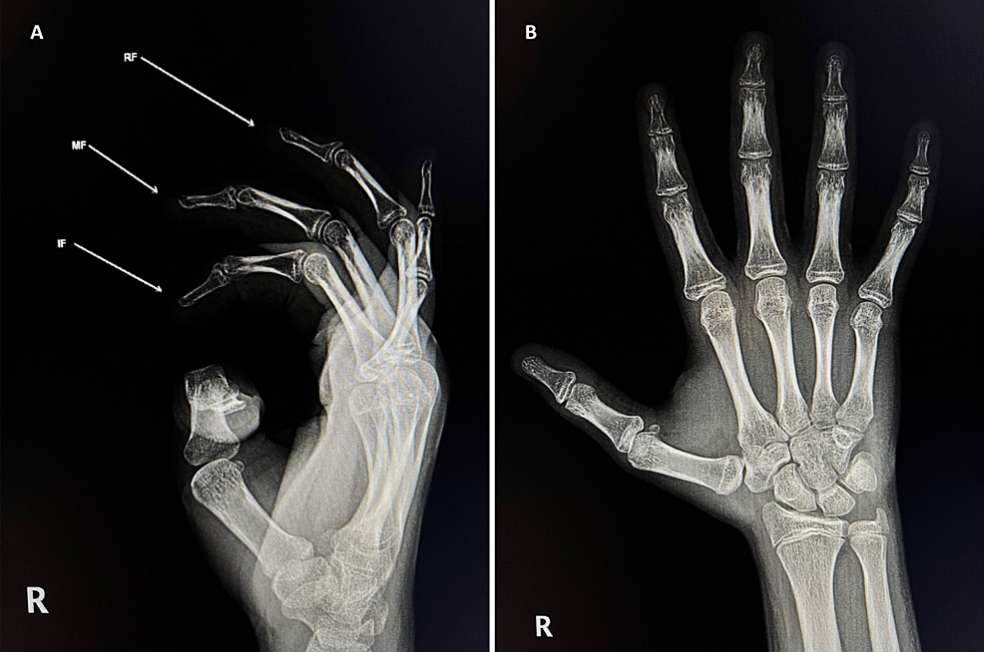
Three-month follow-up x-ray. (A) Lateral view, (B) Anteroposterior view. Abbreviations: IF, index finger; MF, middle finger; RF, ring finger.
